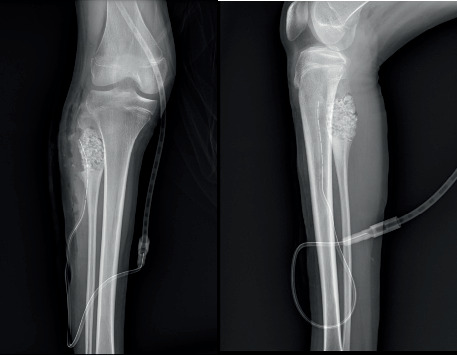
The postoperative plain radiographs demonstrate successful impaction of the allograft.
